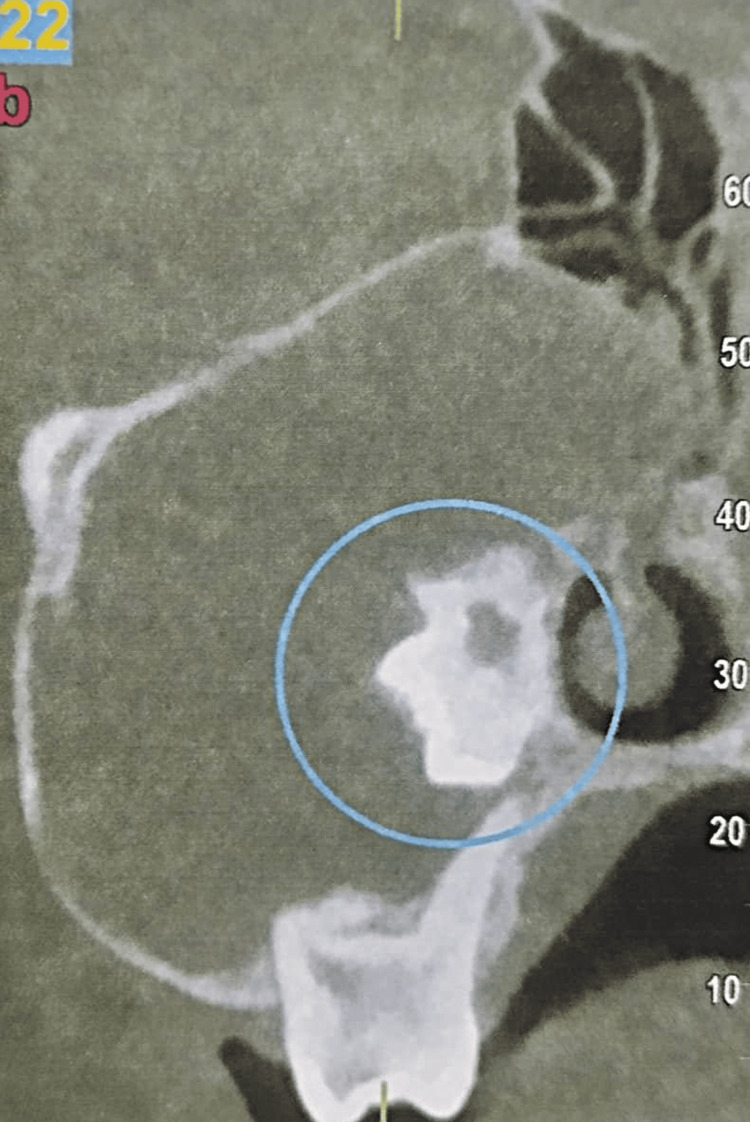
CBCT image showing radiographic evaluation of the ameloblastoma visualized in the scan. CBCT, cone beam computed tomography
